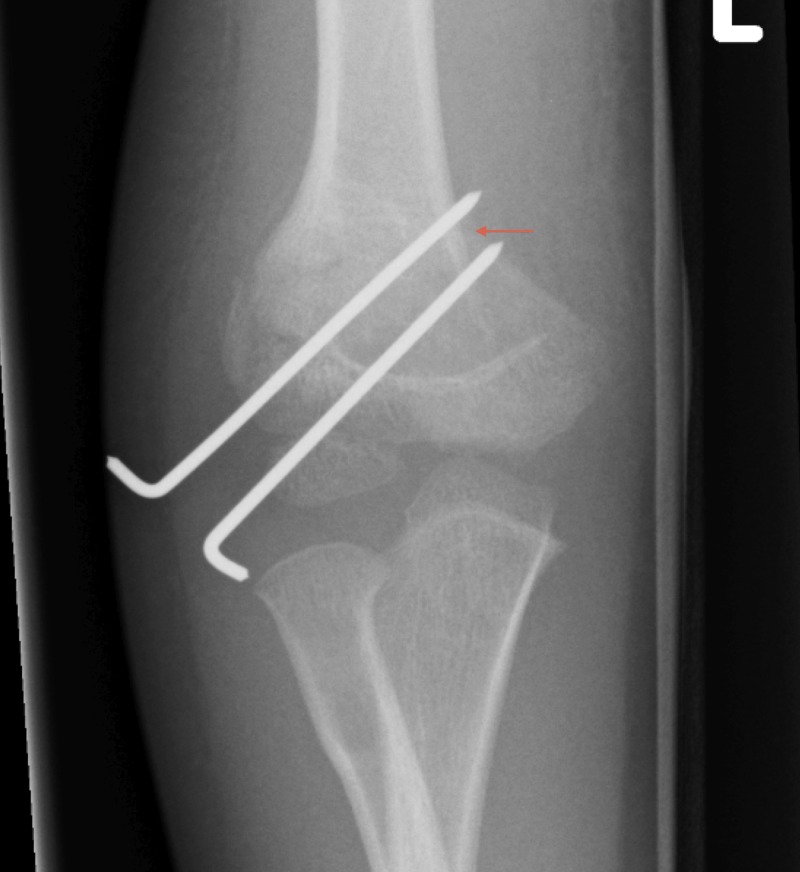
Failure of fixation identified on one-week follow-up radiograph (red arrow).
